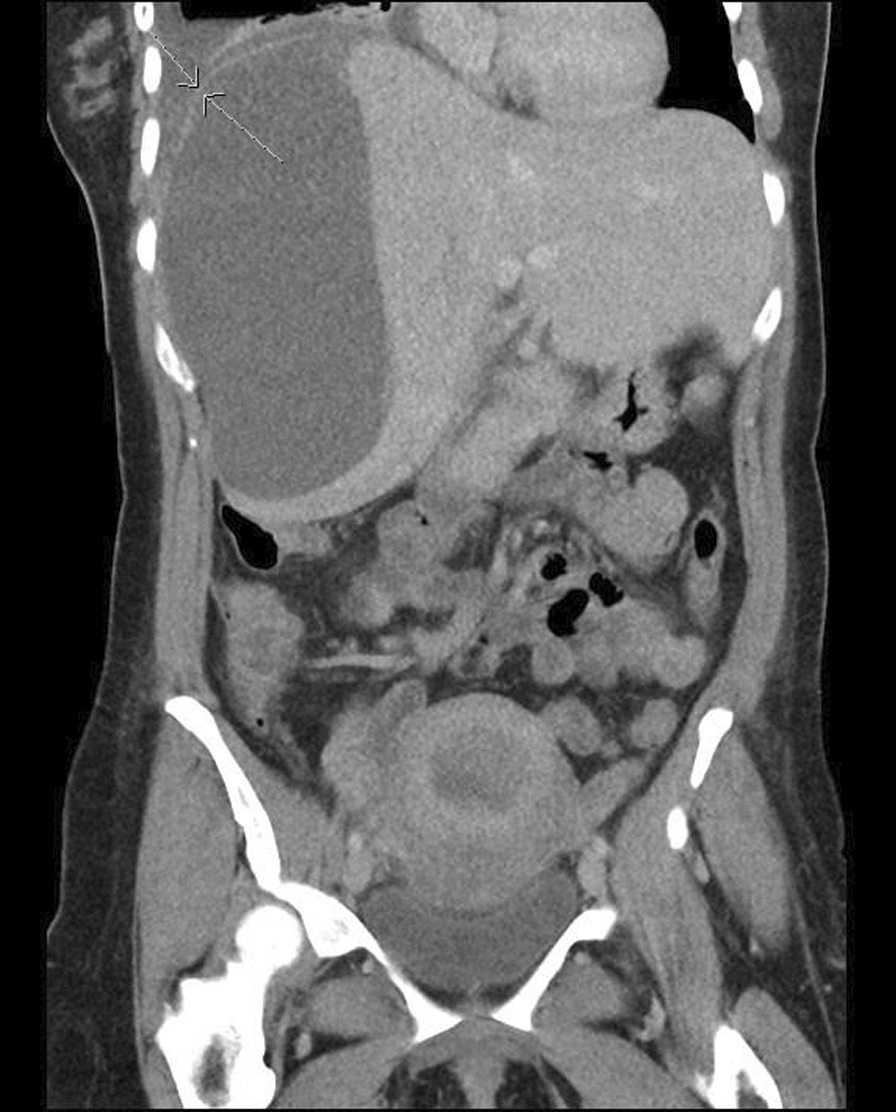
Computed tomography abdomen postoperative day 15: Large subcapsular liver hematoma has increased in size, now measuring 18 cm in cranial-caudal dimension. However, no CT features of active bleed are present. Along the superior margin of the hematoma, the liver capsule demonstrates marked thinning.
